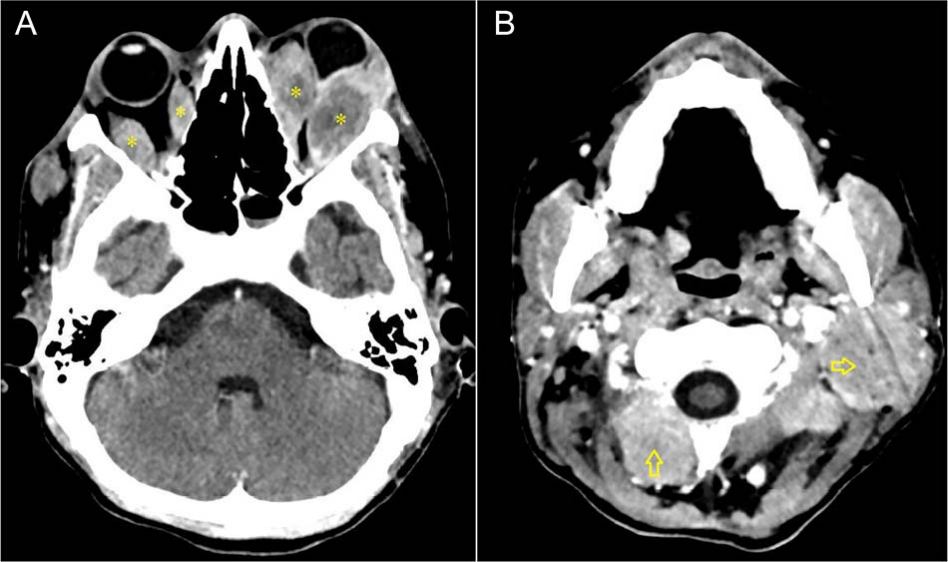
Selected axial cut of the brain CT scan for our patient (A) demonstrates a bilateral asymmetrical fusiform enlargement of the extra-ocular orbital muscles (Asterisks) most appreciated at the lateral rectus muscles and more at the left side, relatively sparing the anterior tendon. Associated with exophthalmos. Neck CT scan selected axial cut (B) Shows bilateral enlarged cervical lymph nodes (Arrows).
